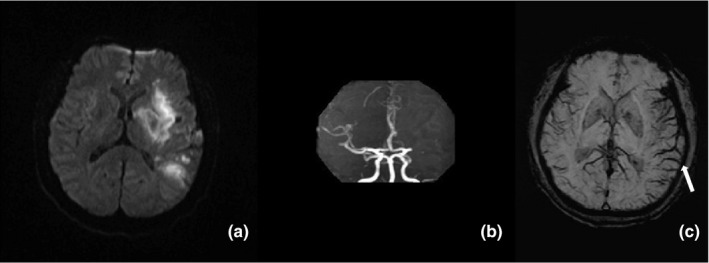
A 60‐year‐old male patient with a history of hypertension and diabetes for 5 years was admitted for AIS 3 hr after onset. He had aphasia, right hemiplegia, and an NIHSS score of 11 on admission. He was treated with IVT 4 hr after onset. An MRI scan was performed 24 hr after onset. DWI showed multi‐focal acute infarcts in the left MCA territory (a); MRA showed occlusion of the left MCA in the M1 segment (b); and SWI showed more and larger vessels with signal loss, indicating ACVS (Grade 3, white arrow) in the left MCA territory (c). His mRS was 4 at 90 days after onset, and the patient remained dependent
